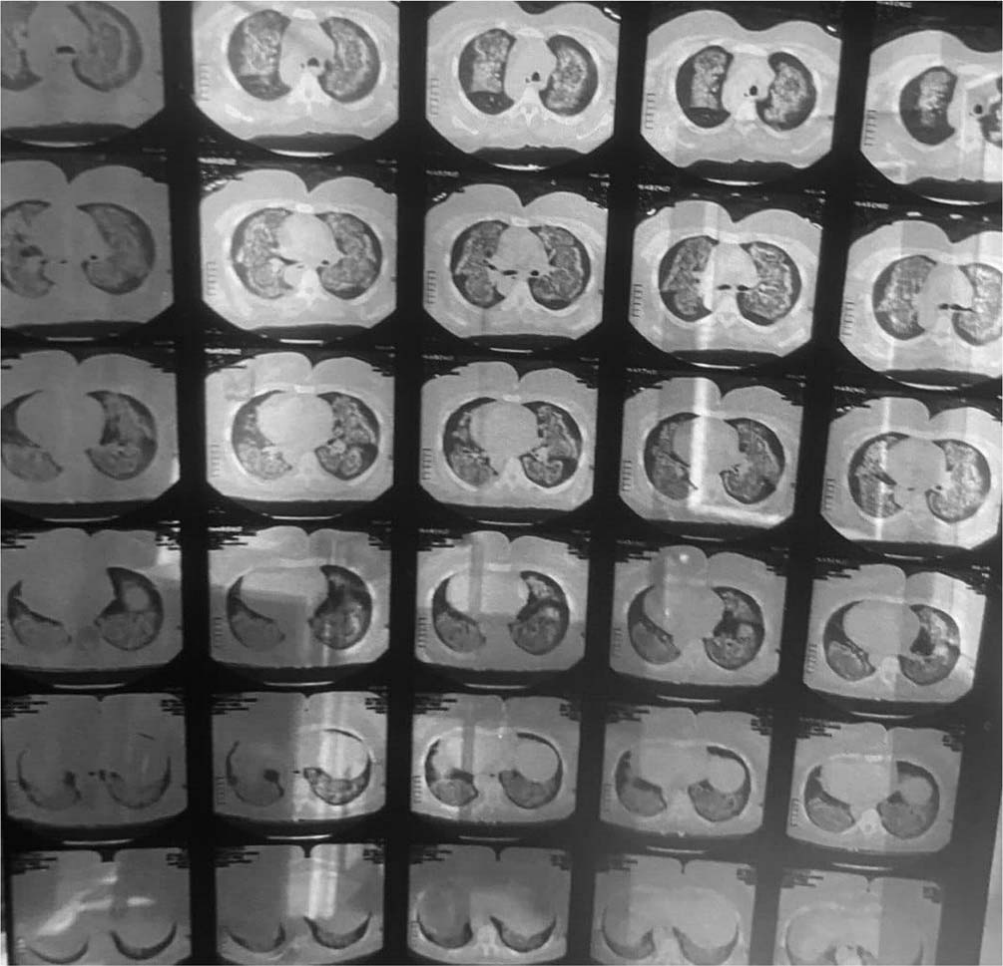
A high-resolution computed tomography (HRCT) of the lungs shows: Diffuse ground-glass opacities, which were highly suggestive of DAH.
